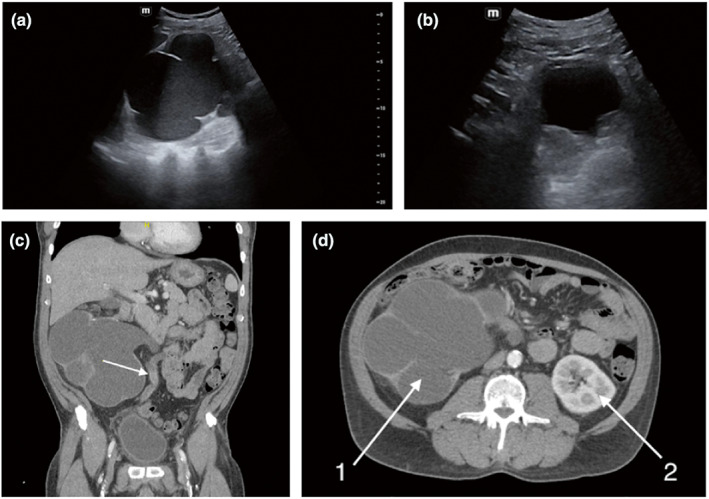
Severe hydronephrosis of the right kidney due to an obstructing bladder mass in a 62 year old man. (a) Point‐of‐care ultrasound (POCUS) demonstrating severe right hydronephrosis with near‐complete obliteration of the renal parenchyma. (b) POCUS of the pelvis showing a bladder with thickened wall. (c) Coronal computed tomography (CT) of abdomen/pelvis showing severe right hydronephrosis and significant right hydroureter (arrow). (d) Axial CT comparing right kidney with severe hydronephrosis (1) with normal left kidney (2).
